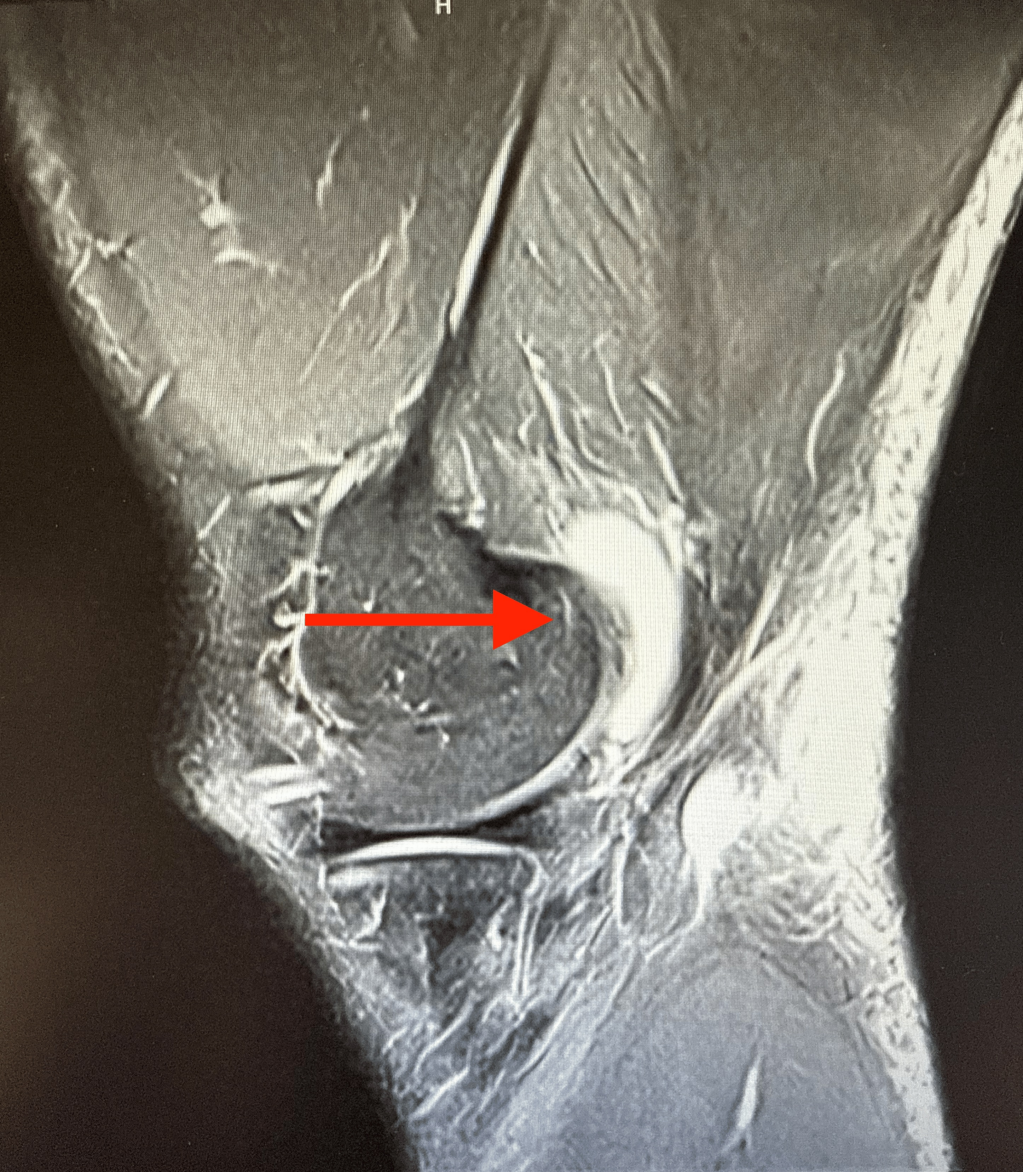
Sagittal MRI showing the position of the wound relative to the distal portion of the semitendinosus at the level of the knee. Collection of fluid and discontinuation of the tendon (arrow).
